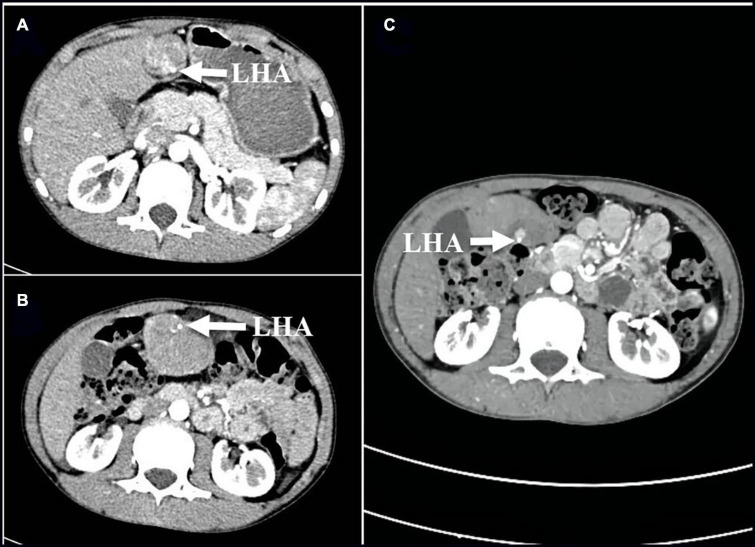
(A,B) Cross-sectional images of the first enhanced CT scan before admission on 25 April 2021 show 180° torsion of the left hepatic artery (arrow); (C) On 27 April 2021, compared with the previous CT scan, the enhanced CT scans repeated after the disappearance of abdominal pain symptoms suggested that the twisted hepatic artery has reset itself (arrows). LHA, left hepatic artery; CT, computed tomography.
